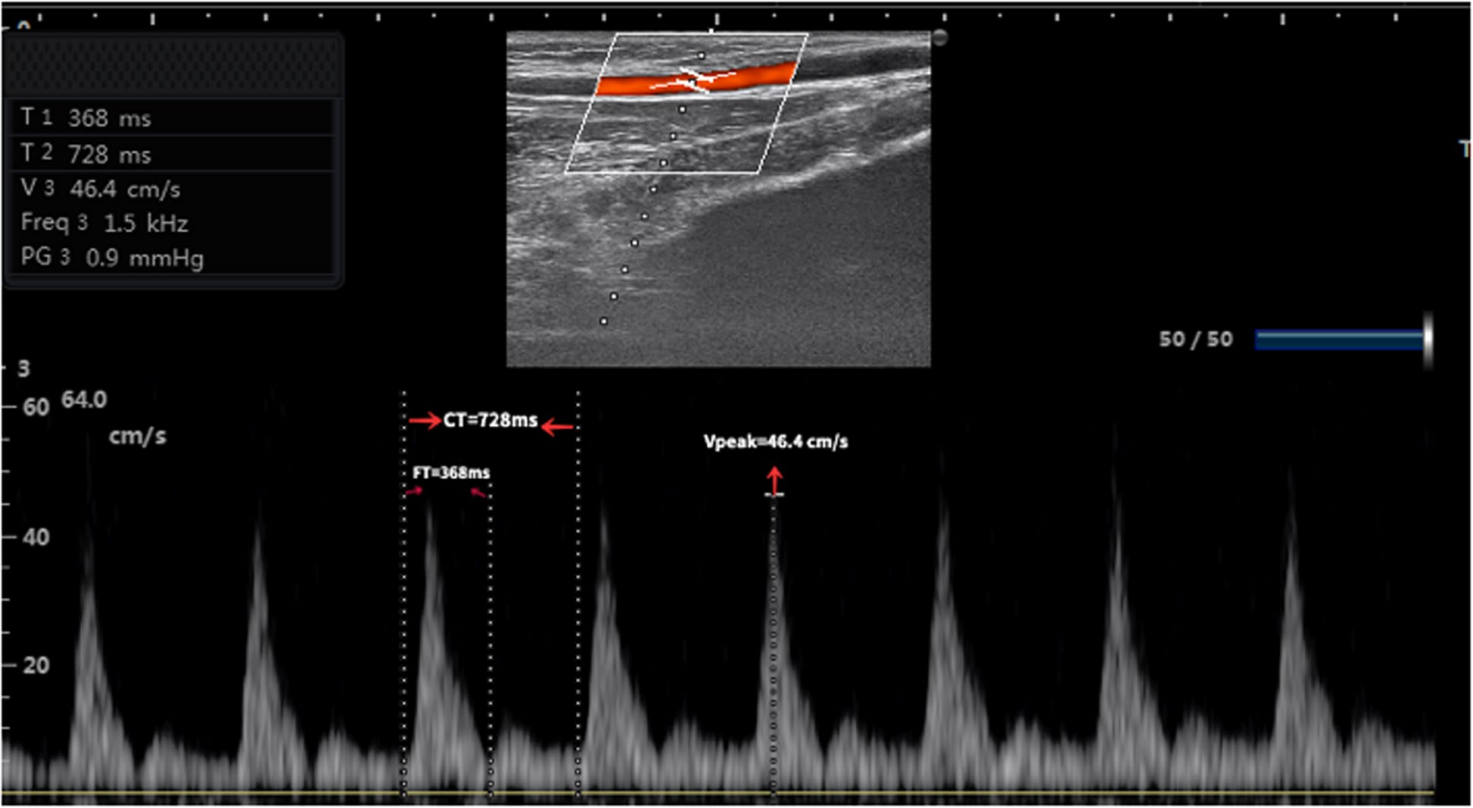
Example ultrasound images of radial artery FT and Vpeak. Radial artery FT and Vpeak were measured adjacent to the radial head. FTc was calculated by using a simplified formula FTc = FT+ [1.29 x (HR - 60)] [12]. ΔVpeak was calculated as follows: (maximum peak velocity-minimum peak velocity) / [(maximum peak velocity + minimum peak velocity)/2]× 100 [10]. FT: radial artery flow time; Vpeak: radial artery blood flow peak velocity
